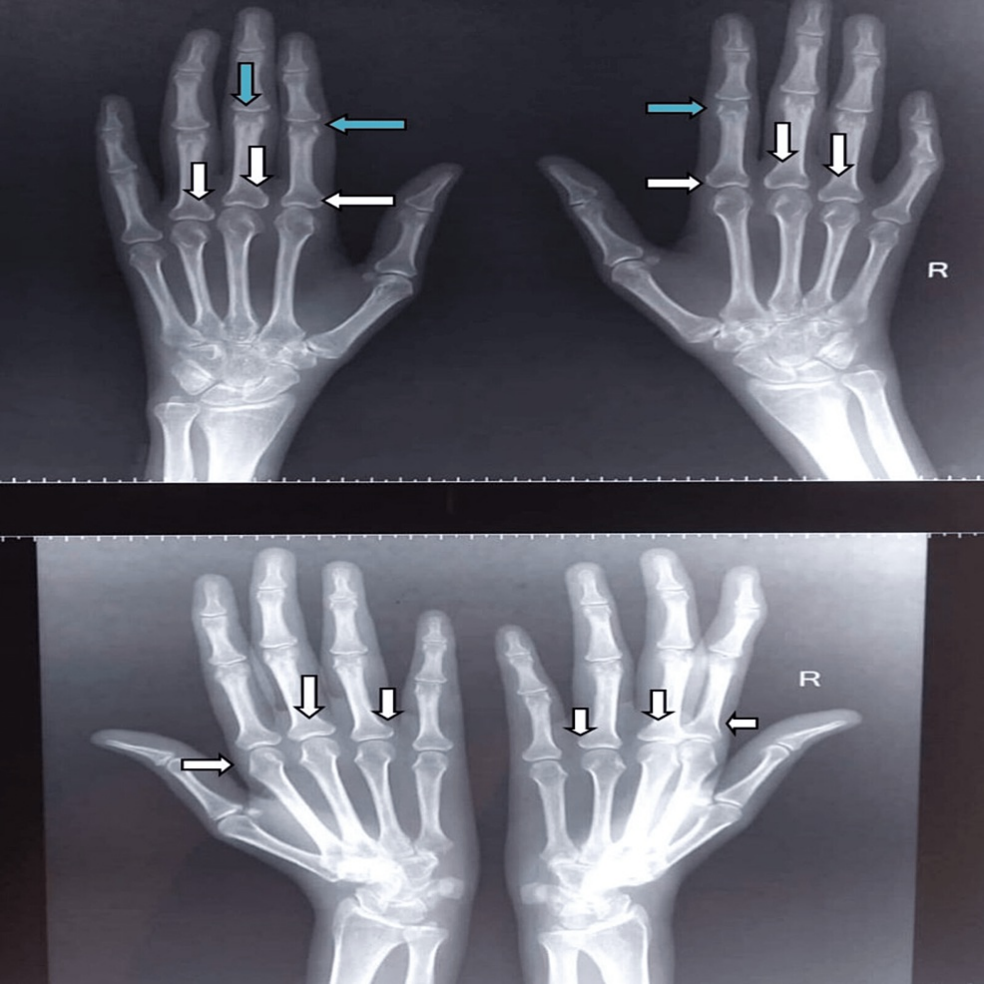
X-ray showing juxta-articular osteopenia and periarticular erosions in proximal interphalangeal joints (blue arrow) and metacarpophalangeal joints (white arrow).
